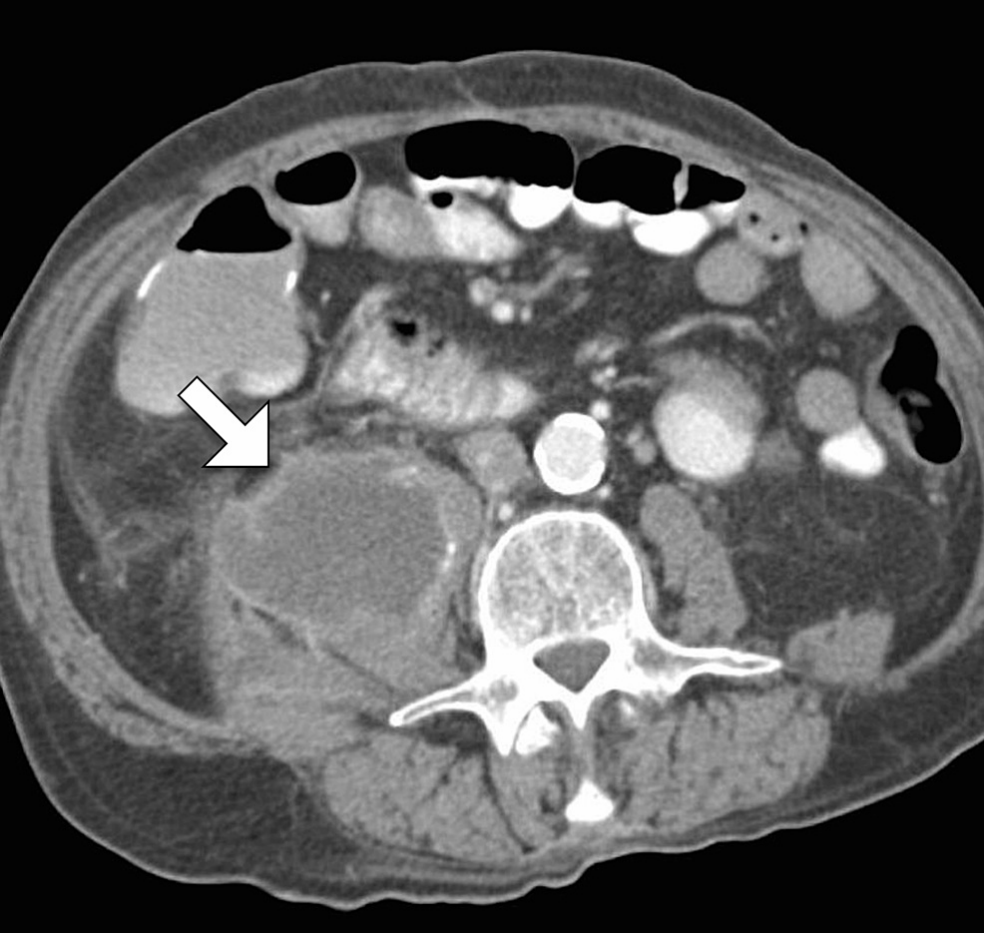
Axial CT image of the abdomen reveals a collection within the right psoas muscle (arrow) with a thickened enhancing wall, consistent with an abscess CT: computed tomography
